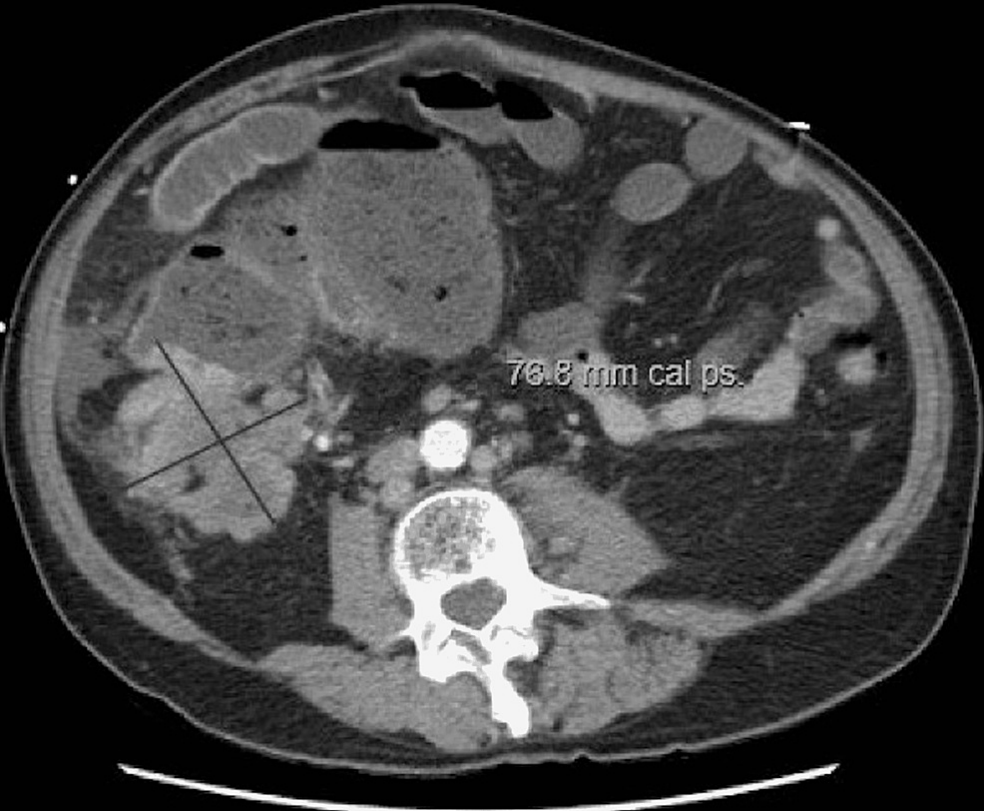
CT abdomen and pelvis showing irregular exophytic soft tissue mass within the ascending colon, with enlarged retroperitoneal lymph nodes
